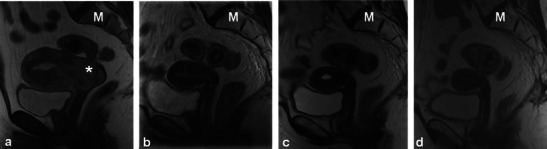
A 42-year-old woman with cervical squamous cell carcinoma showing expected post-RT findings over consecutive MRI examinations. Sagittal T2 TSE sequences performed at baseline (a) and 1-year intervals thereafter (b,c and d). Baseline imaging a demonstrates extensive tumour centred in the cervix with uterine and vaginal invasion (*asterisk*), and malignant obstruction of the cervical os causing hydrometra. Parametrial invasion was also present (not shown). Note normal marrow signal intensity (*M*). One-year follow-up imaging b demonstrates reconstitution of the normal zonal anatomy of the cervix and presence of homogeneous low signal intensity of the cervical stroma reliable indicators of complete response. Note global uterine atrophy and fatty replacement of the pelvic marrow, which are expected imaging findings following pelvic RT. Subsequent follow-up examinations performed 2 (c) and 3 (d) years after therapy show progressive uterine atrophy but no recurrent tumour
